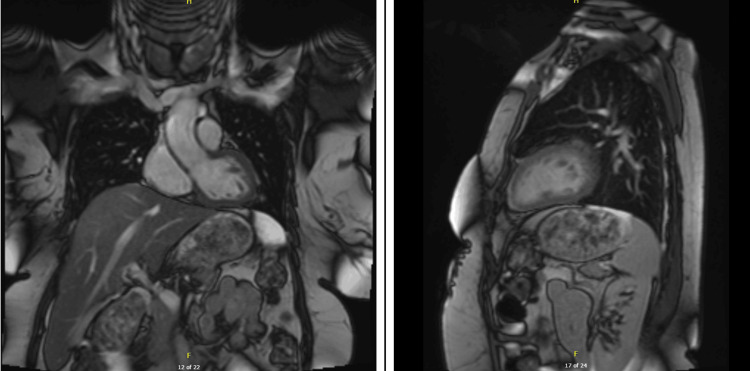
Follow-up cardiac magnetic resonance imaging (MRI) on day seven demonstrating functional recovery and absence of myocardial injury. Cardiac MRI performed on day seven demonstrated normalized left ventricular (LV) volumes (end-diastolic volume (EDV): 97 mL; indexed EDV: 54 mL/m²) and ejection fraction (EF) of 75%. There was no late gadolinium enhancement (LGE), ruling out myocardial infarction or fibrosis. A small area of isolated peri-myocardial enhancement was noted in the inferior basal wall, likely representing reactive changes. T1 and T2 parametric mapping values were within normal limits (T1 (septal): 1092 milliseconds; T2: 47 milliseconds), effectively ruling out myocarditis or infarction and supporting the diagnosis of stress-induced (takotsubo) cardiomyopathy.
